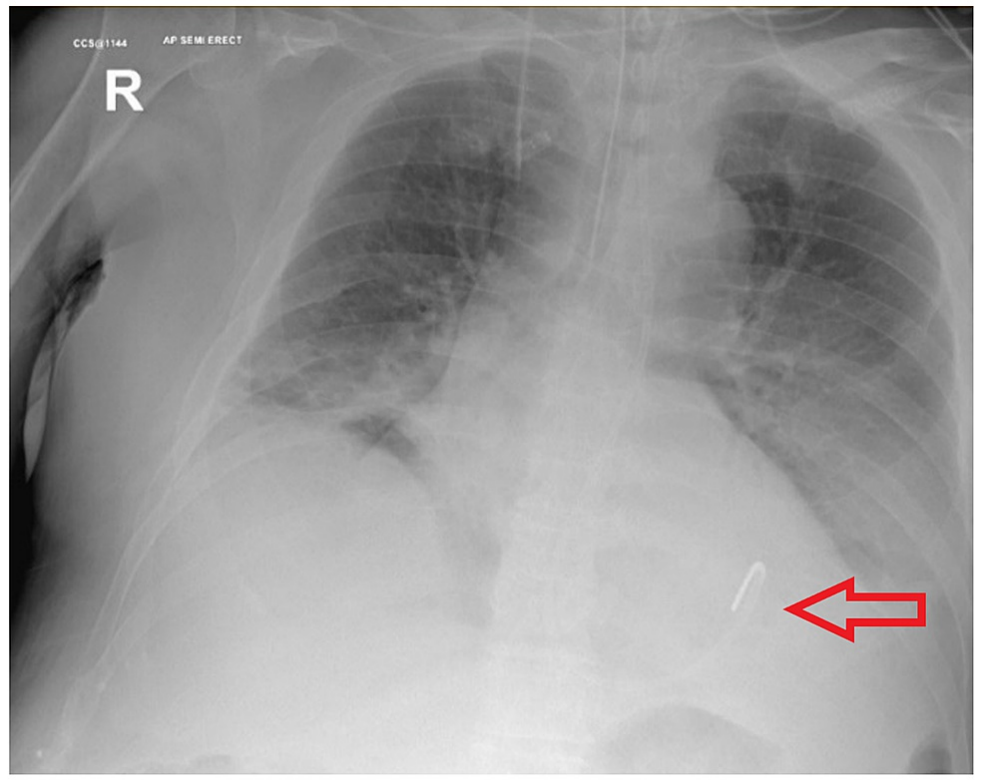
Post-placement x-ray confirming a satisfactory position.
